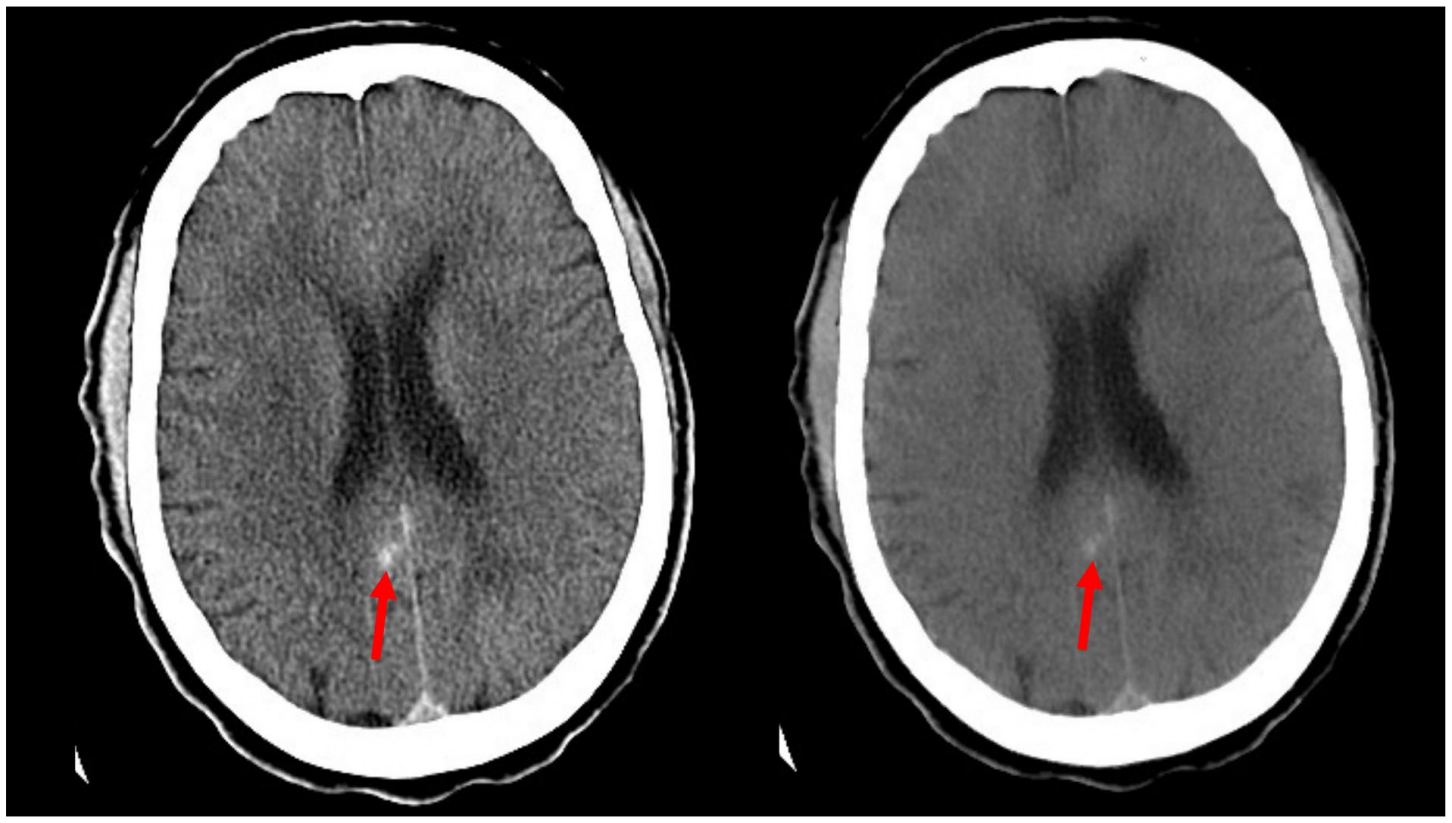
Example of a false negative reported by the Aidoc algorithm: a small area of hemorrhage along the posterior cingulate gyrus (red arrows) as seen in the contrast CT (left) is confirmed by persistence in the iodine suppression image (right).
